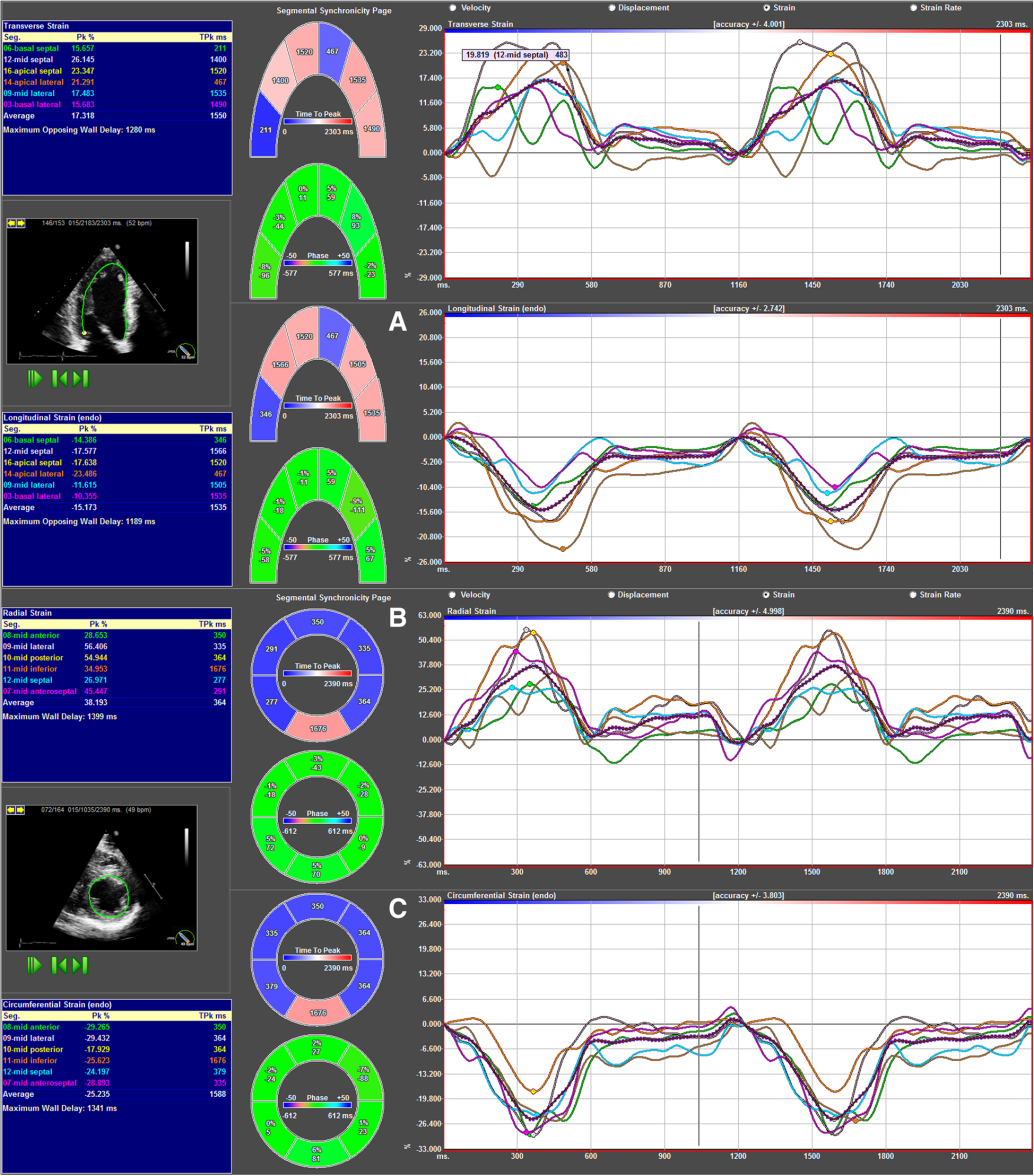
Representative layout of the speckle tracking analysis in an athlete. Using the apical four-chamber view (upper part) and the midpapillary level of the parasternal short-axis view (lower part), negative values of longitudinal strain (A), positive values of radial strain (B) and negative values of circumferential strain (C) of the corresponding segments can be obtained.
